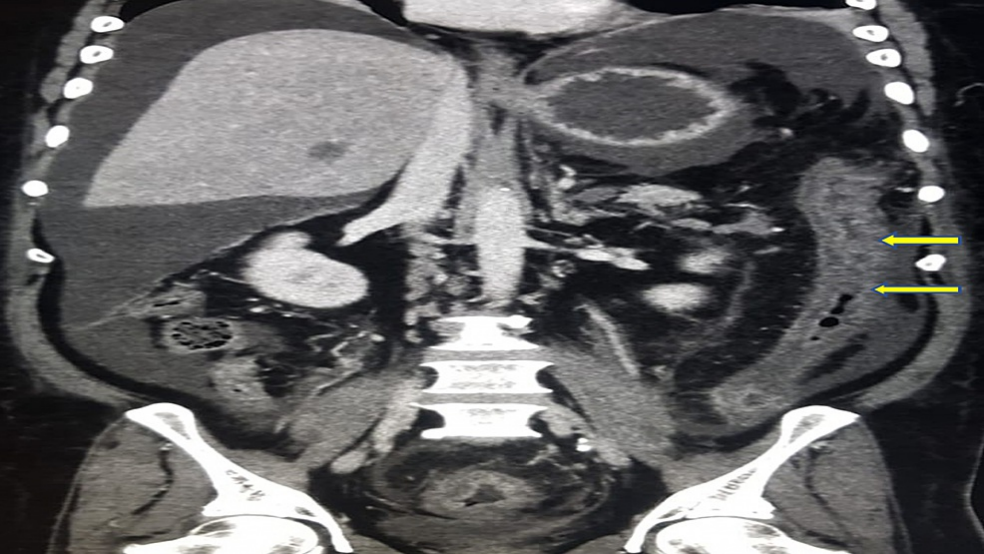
Abdominal computed tomography with contrast (coronal view) showing decreased mural enhancement with mural thickening and luminal narrowing of the descending colon (yellow arrows).
